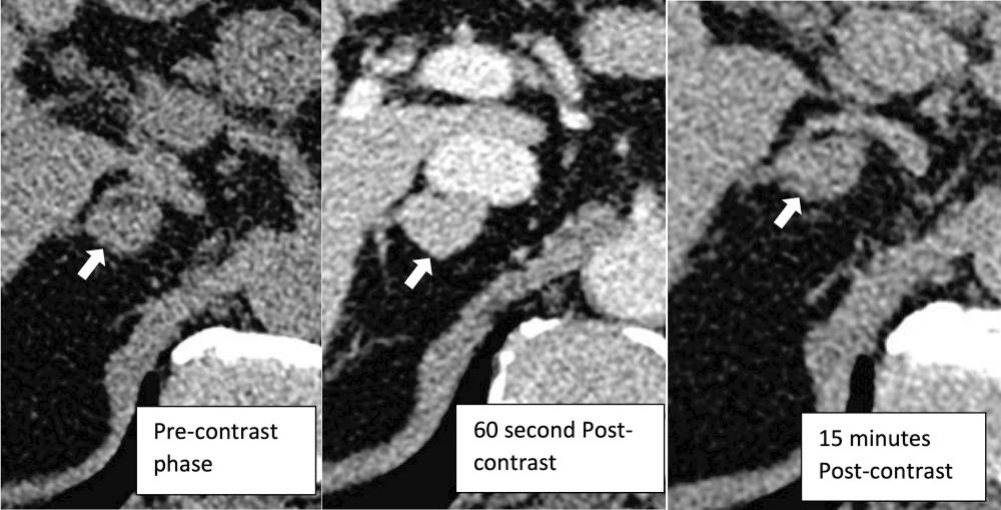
Contrast-enhanced CT adrenal showing a 2.0 cm × 1.3 cm homogenously enhancing right adrenal lesion (white arrow).
